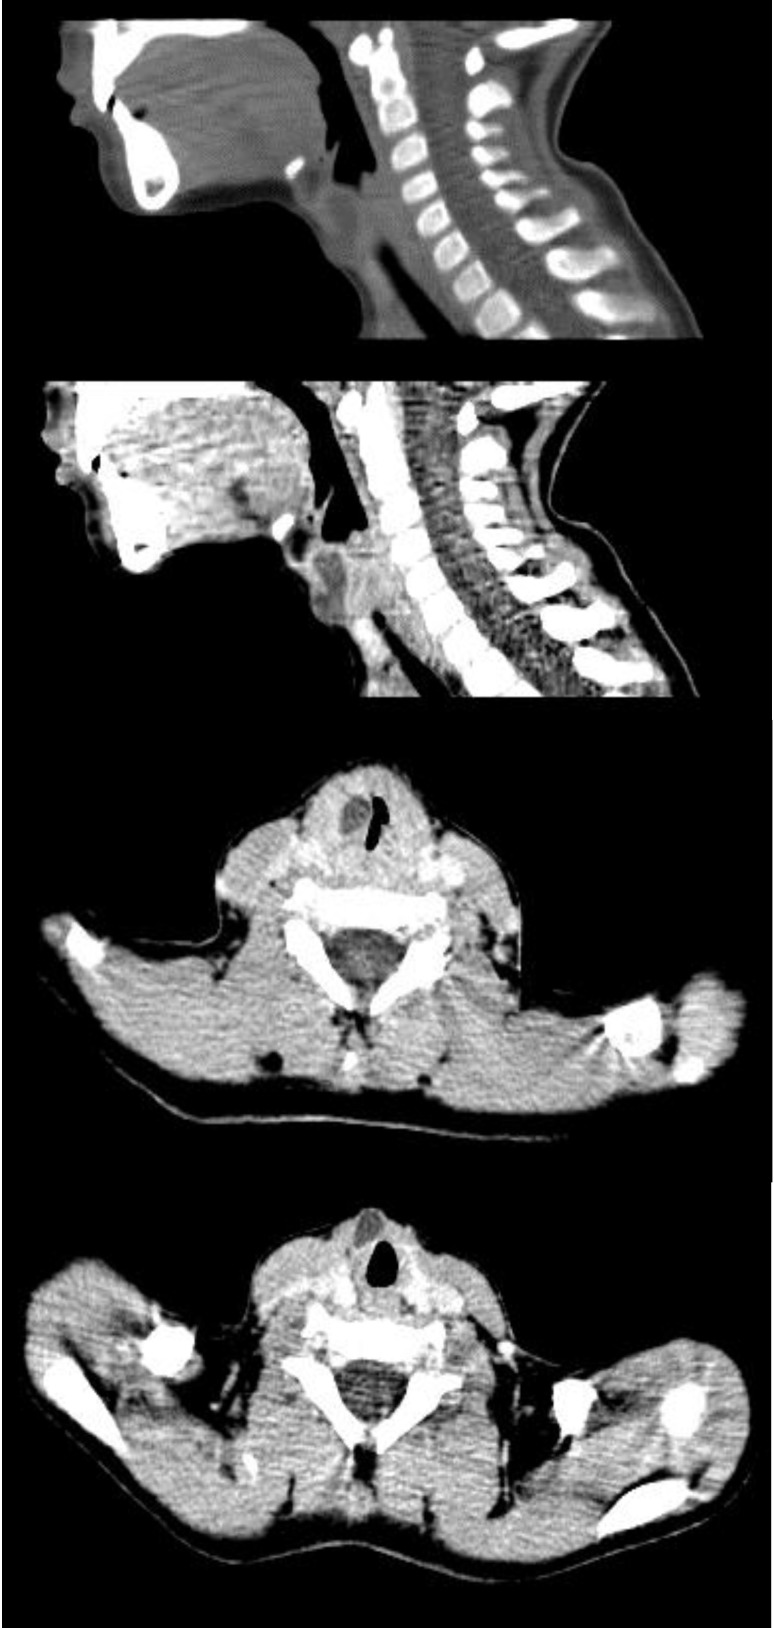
CT-scan of the patient, showing a laryngeal cyst extending through the cricothyroid membrane into the cervical region
